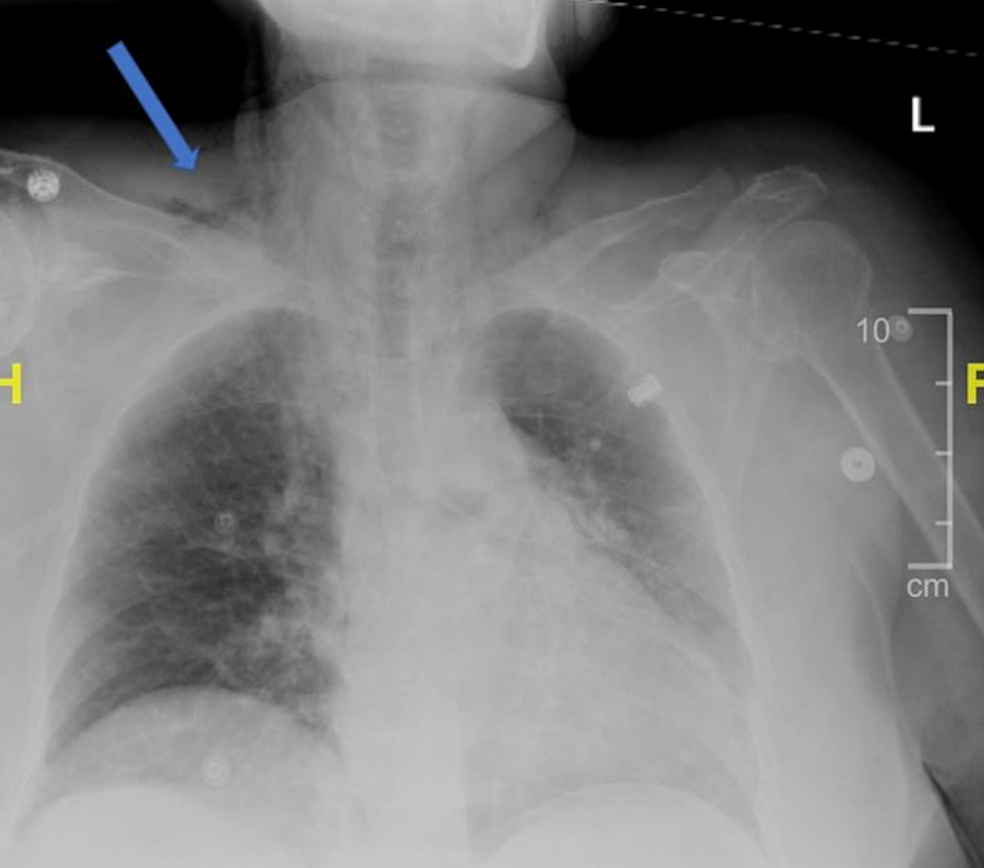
Chest x-ray showing subcutaneous emphysema (blue arrow)
